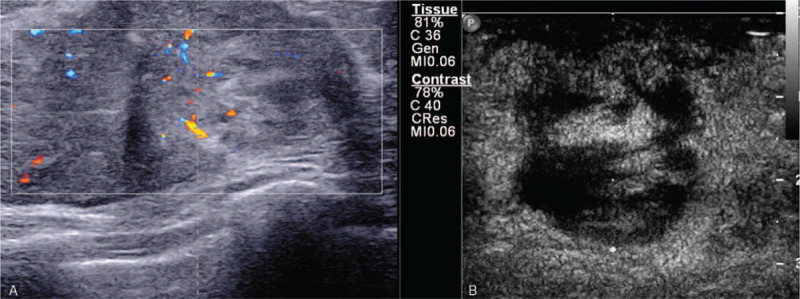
(A) B-mode and color Doppler ultrasound images of the left breast showed a diffuse inhomogeneous isoechoic, well-defined lesion and blood vessels within the lesion. (B) Contrast-enhanced ultrasound images showed the mass an extensive area of perfusion defects.
